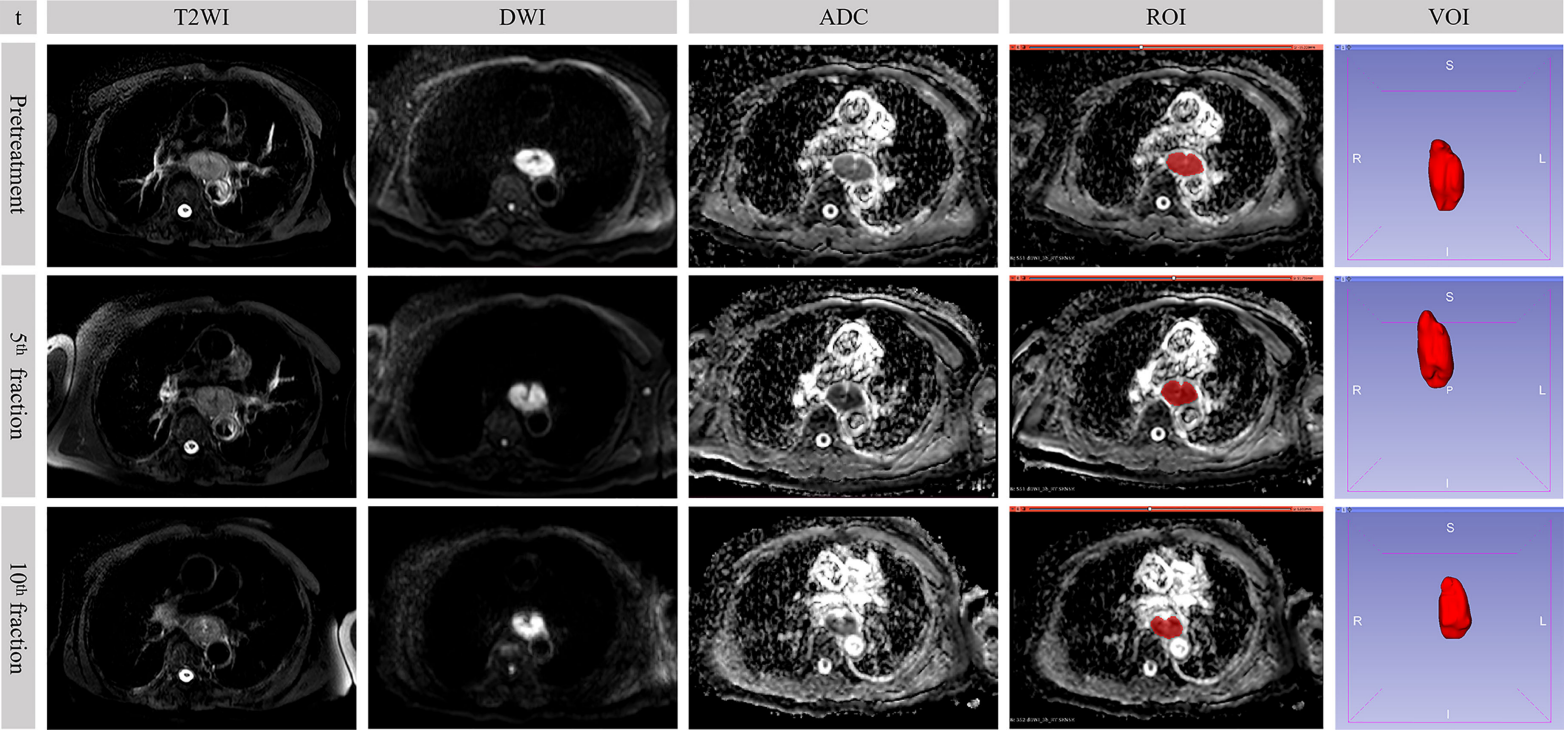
Sequential MR images in a 73-year-old male who underwent concurrent chemoradiotherapy with partial response. The value of *b*-factor of DWI in the figure was 600 s/mm^2^. t, time point; DWI, diffusion-weighted imaging; ADC, apparent diffusion coefficient; ROI, region of interest; VOI, volume of interest.
